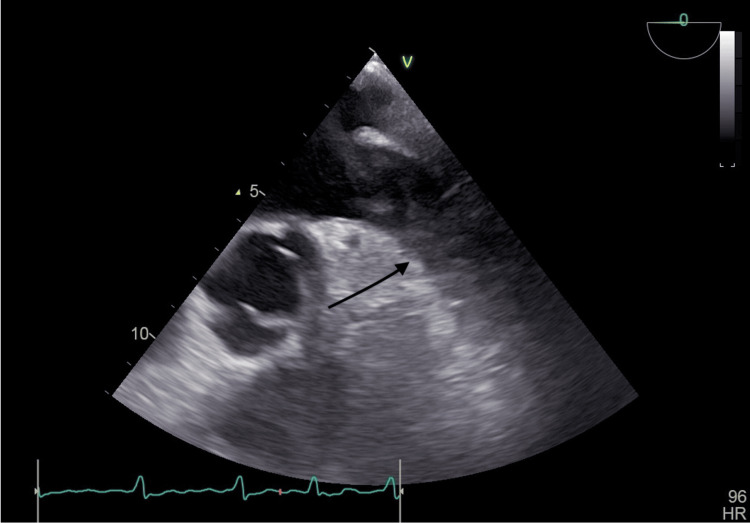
TOE midesophageal 0 degree showed presence of mobile and huge LAA clot (shown by black arrow) about one third of LAA. There was presence of spontaneous echo contrast and freely mobile LAA thrombus. The LAA opening orifies diameter was dilated measured 3.4 cm. TOE, transesophageal echocardiography; LAA, left atrial appendage.
